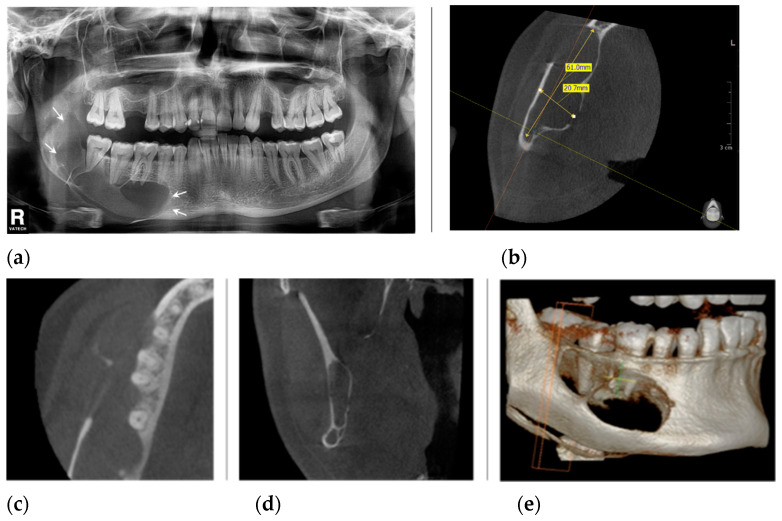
The radiological aspect before surgical treatments: (a) panoramic radiograph shows a large multilocular lesion occupying the body of the mandible, from the right second premolar to the ascendent ramus (arrows), without involving the condyle or coronoid process, and inferior displacement of the mandibular canal; (b) the CBCT, axial view shows the lesion measuring 61 mm in length and 20.7 mm in width; (c) the CBCT image indicates that the apices of the first and second molar are in the unaffected bone structure; (d) the CBCT, coronal view of the ascending ramus, indicates the condyle is not involve; (e) the CBCT, 3D reconstruction view.
